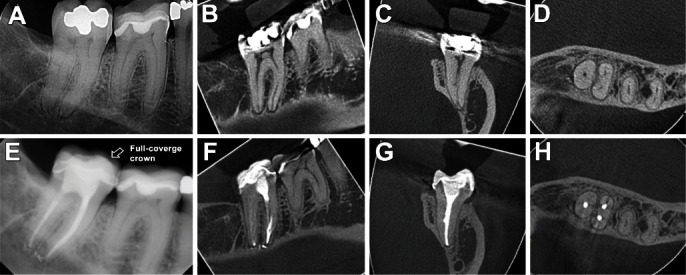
Initial digital radiography and CBCT images before treatment of tooth #31 (A–D); radiography and CBCT follow-up at 2 years, respectively (E–H).
